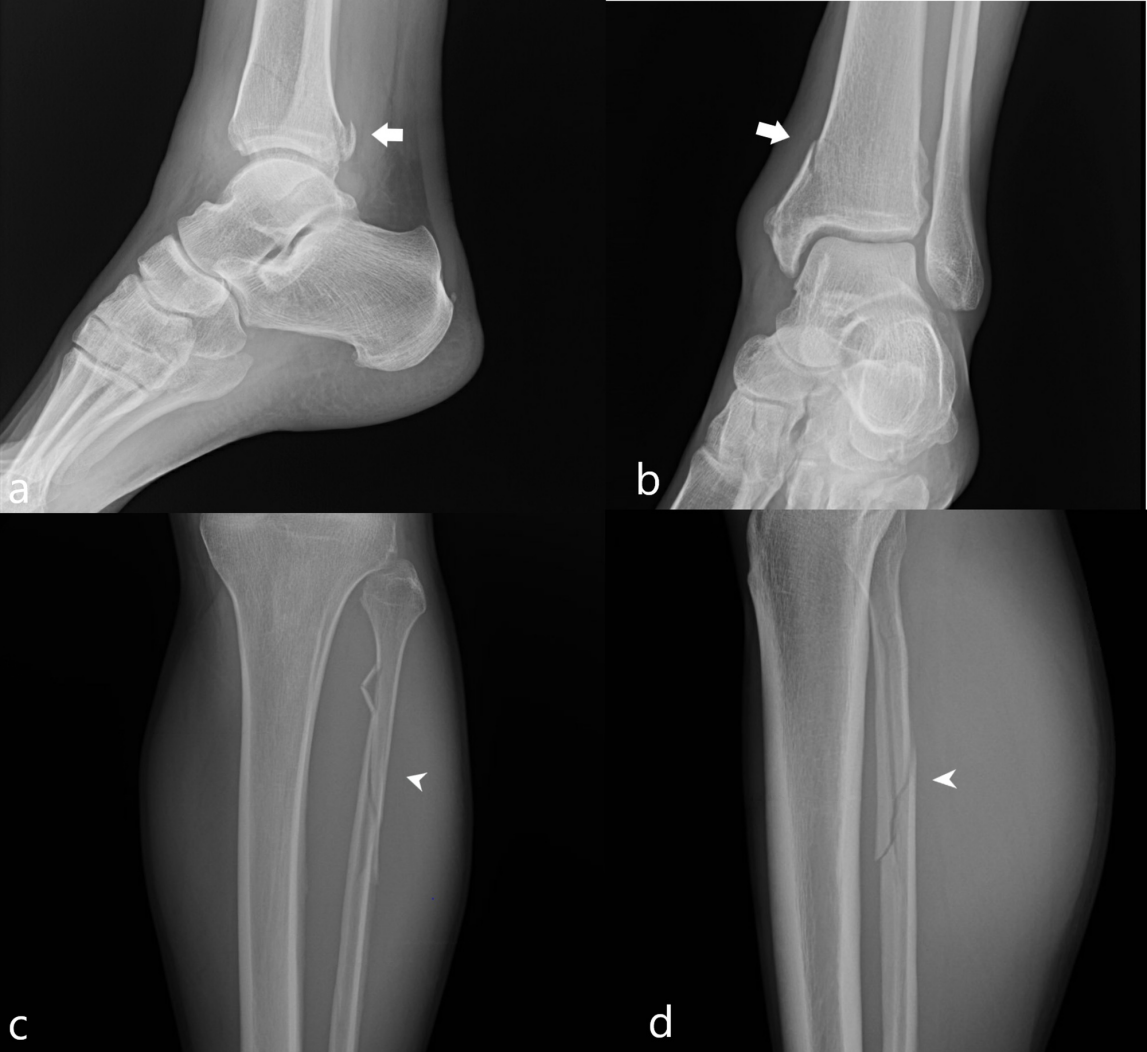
Case 10 radiographic images of the ankle and tibia/fibula A lateral radiograph of the ankle (a) demonstrates a mildly displaced fracture of the posterior malleolus (white arrow). An oblique mortise view radiograph of the ankle (b) demonstrates a nondisplaced fracture of the medial malleolus (white arrow). Frontal (c) and lateral (d) radiographs of the tibia demonstrate a spiral fracture of the proximal fibula (white arrowhead). The constellation of findings is consistent with a Maisonneuve fracture.
